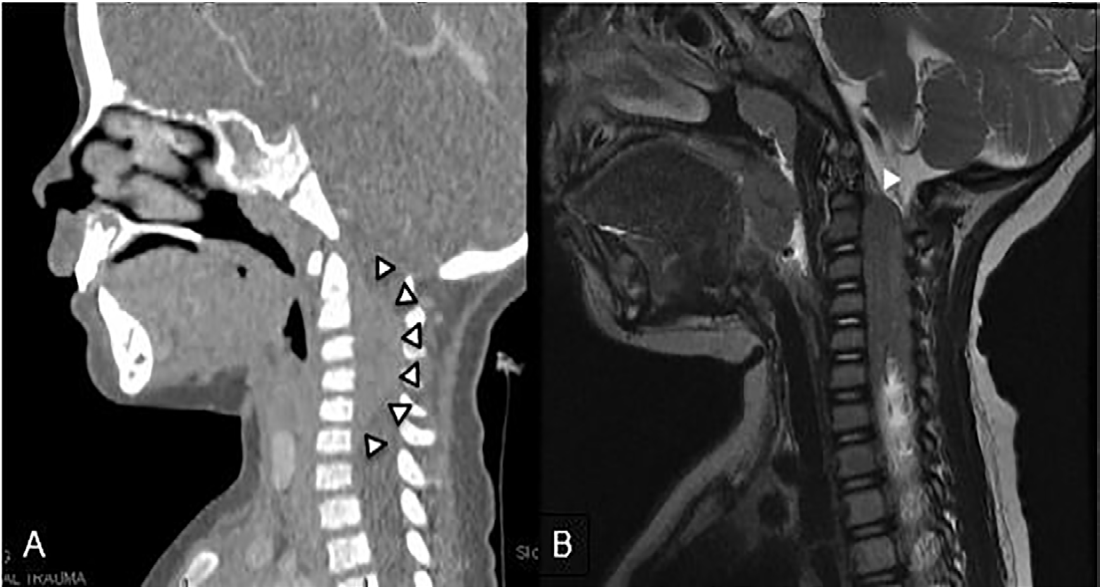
(A) Computed tomography with contrast of neck soft tissue, initially read as containing no pathology. Note the subtle hyperlucent lesion extending from the level of the second cervical vertebra (C2) to the seventh cervical vertebra (C7) (black-edged white arrows), corresponding to the hypodense region on the magnetic resonance imaging (MRI) (B). MRI with contrast of the cervical spine showing a heterogeneously enhancing mass extending from C2 to C7 with edema and cord flattening (white arrow).
